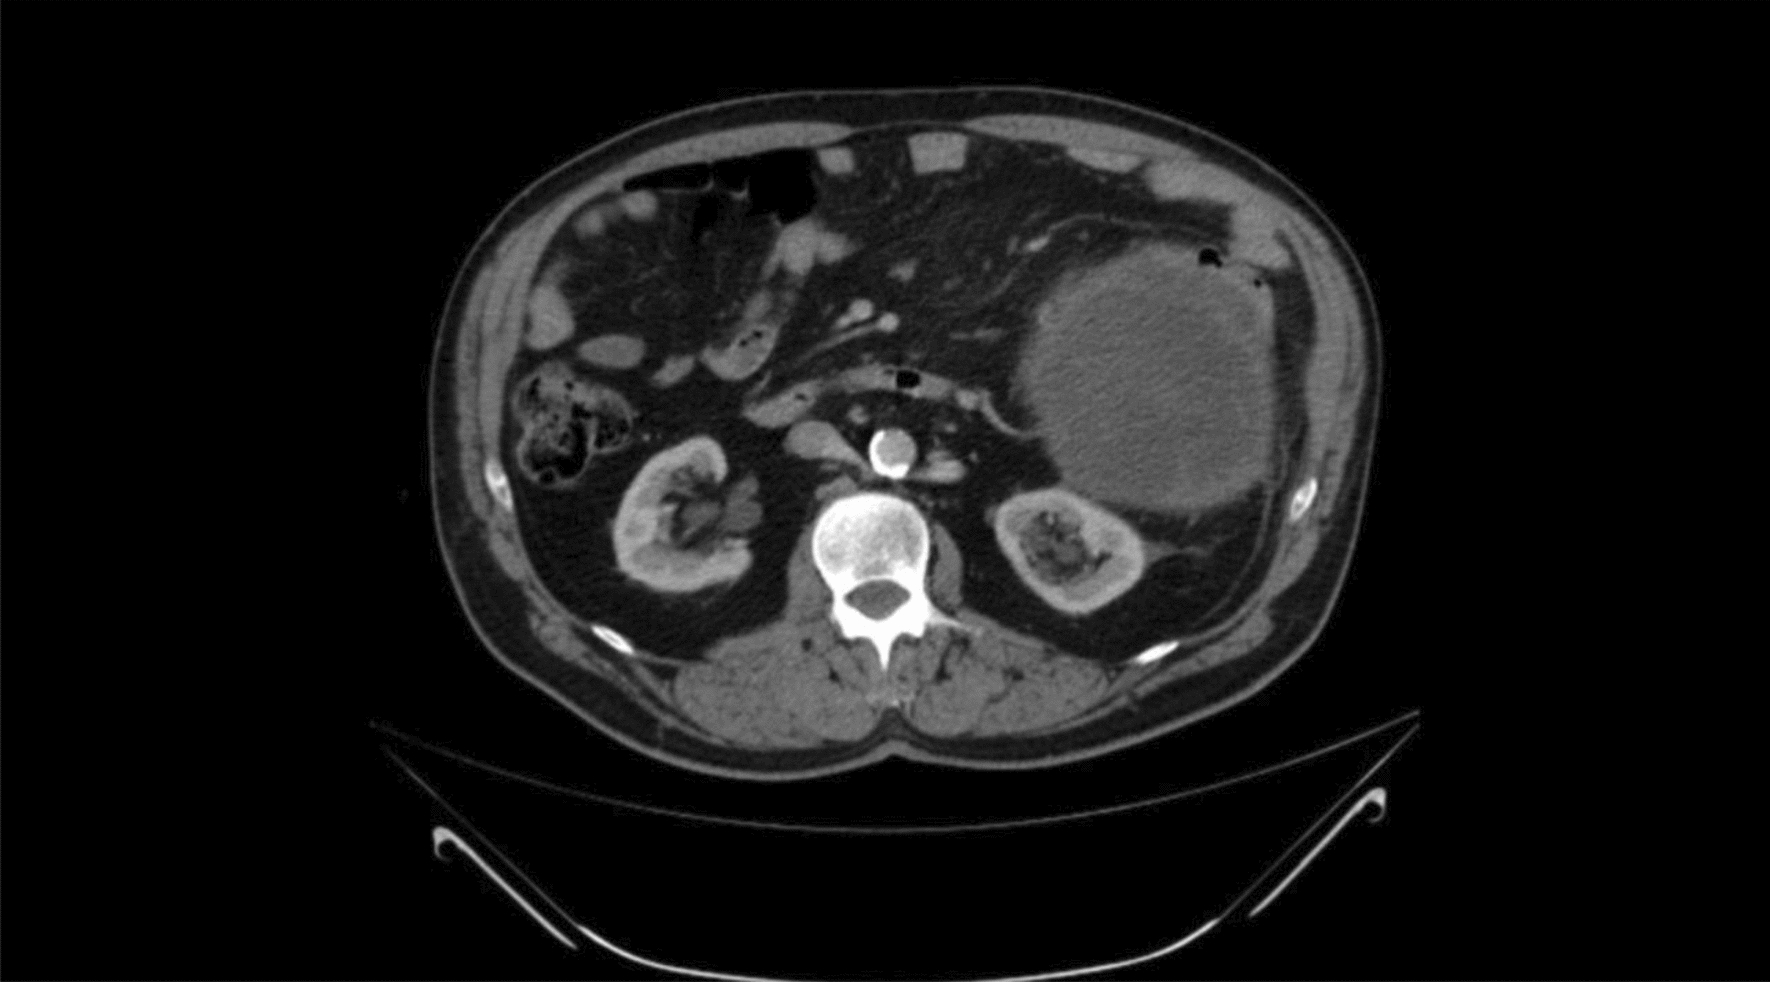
Axial abdominal computed tomography postcontrast study (portal phase) showing large, well-defined lesion with mass effect over the the lower descending colon, pushing it
